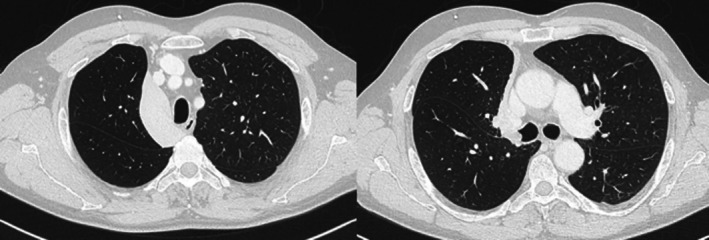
Chest CT scan performed at admission. Chest CT at admission shows right upper lobe atelectasis (left panel) with an endobronchial obstructing lesion in the right upper lobe bronchus (right panel).
